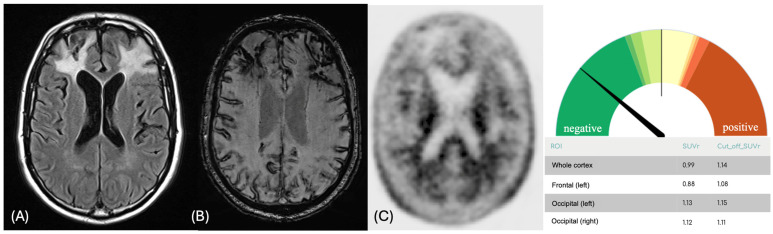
Brain MRI and Amyloid PET of the patient. (A) FLAIR and (B) SWI sequences of the patient in 2017, which showed extensive bifrontal ICHs with massive disseminated cortical superficial siderosis. (C) Amyloid PET tracer uptake ([^18^F]Florbetapir) was reduced in the frontal lobes due to previous lobar ICH. The exam was visually reported as negative for amyloid accumulation, but the semi-quantitative analysis showed borderline SUVr values in the occipital lobes. The Gauge plot provides a graphical representation of the semi-quantitative whole-brain tracer uptake. Semi-quantitative analysis was performed using DOlab Research Version (Dorian Technologies S.r.l.), with regional SUVr values normalized to the cerebellar parenchyma (whole brain SUVr threshold for positivity: 1.14). Legend: ICH = intracerebral hemorrhage; PET = Positron Emission Tomography; SUVr = Standardized Uptake Value ratio.
